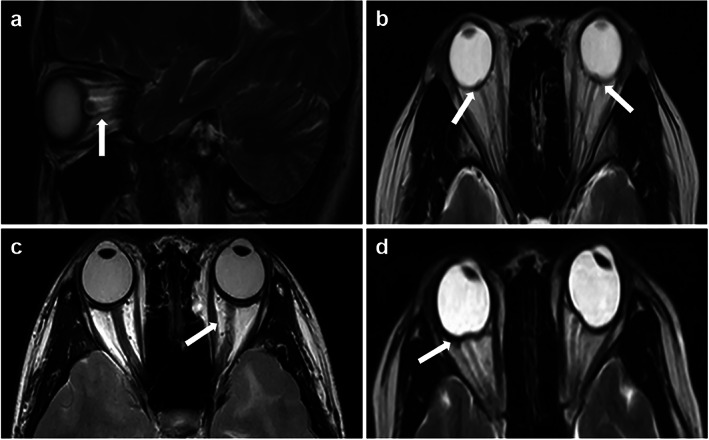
Classical magnetic resonance imaging (MRI) signs suggestive of orbital findings with idiopathic intracranial hypertension (IIH). (a) Sagittal T2‐weighted MRI showing dilation of optic nerve sheath (arrow). (b) Axial T2‐weighted MRI showing a swollen optic nerve head (arrows). (c) Axial T2‐weighted MRI showing tortuosity of the optic nerves (arrow). (d) Axial T2‐weighted MRI showing posterior globe flattening (arrow).
